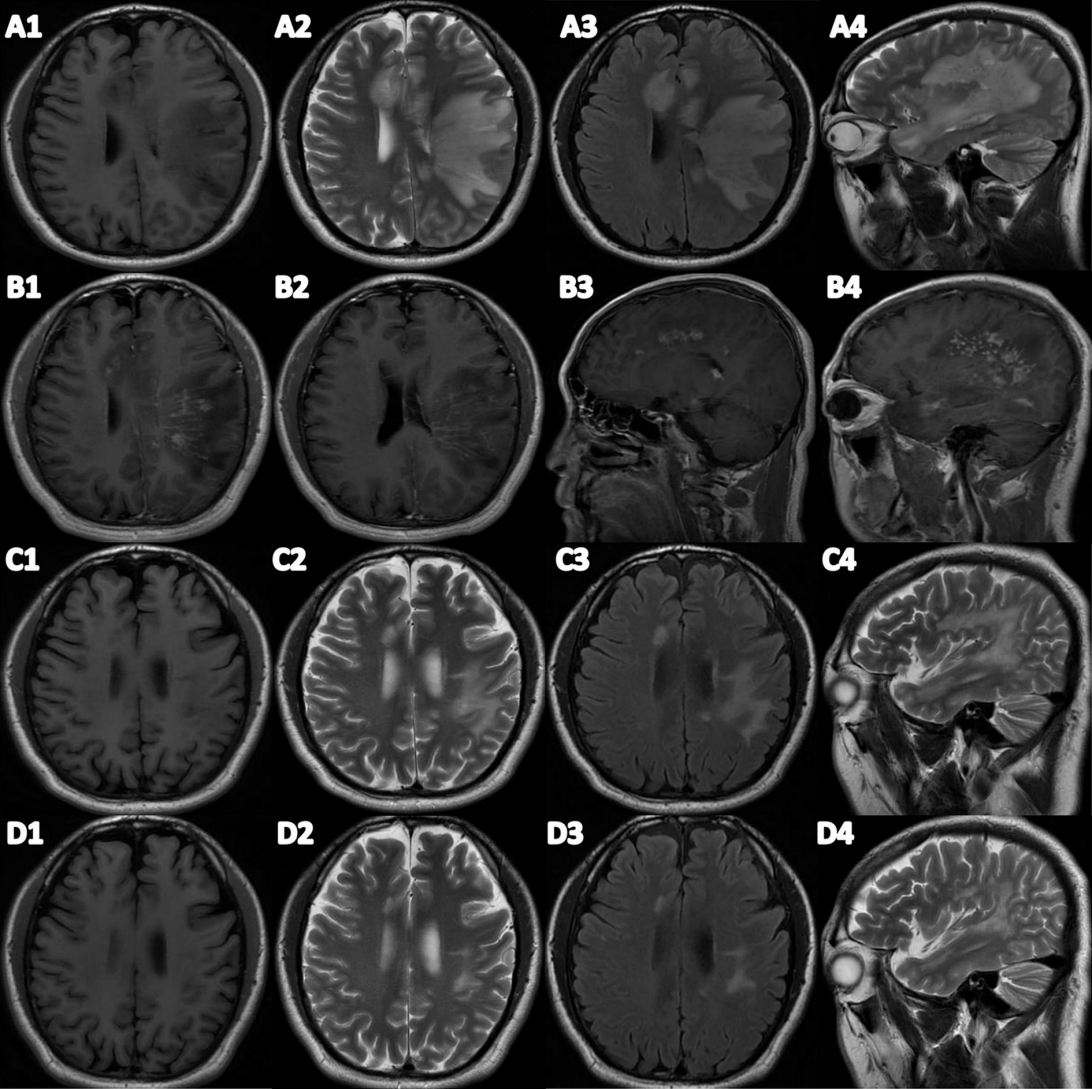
(A1–A4) Brain plain MRI before treatment. (B1–B4) Brain T1-enhanced MRI. (C1–C4) Brain plain MRI after treatment. (D1–D4) Brain plain MRI after 3 months of treatment. (A1/C1/D1) Transverse T1 weight imaging. (A2/C2/D2) Transverse T2 weight imaging. (A3/C3/D3) Fluid attenuated inversion recovery (FLAIR). (A4/C4/D4) Sagittal T2 weight imaging.
